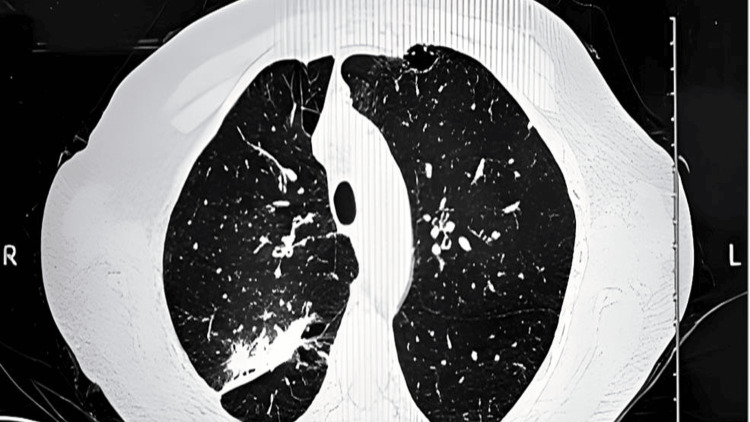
Axial CT chest image demonstrated a mass-like irregular consolidation in the upper lobe of the right lung.
